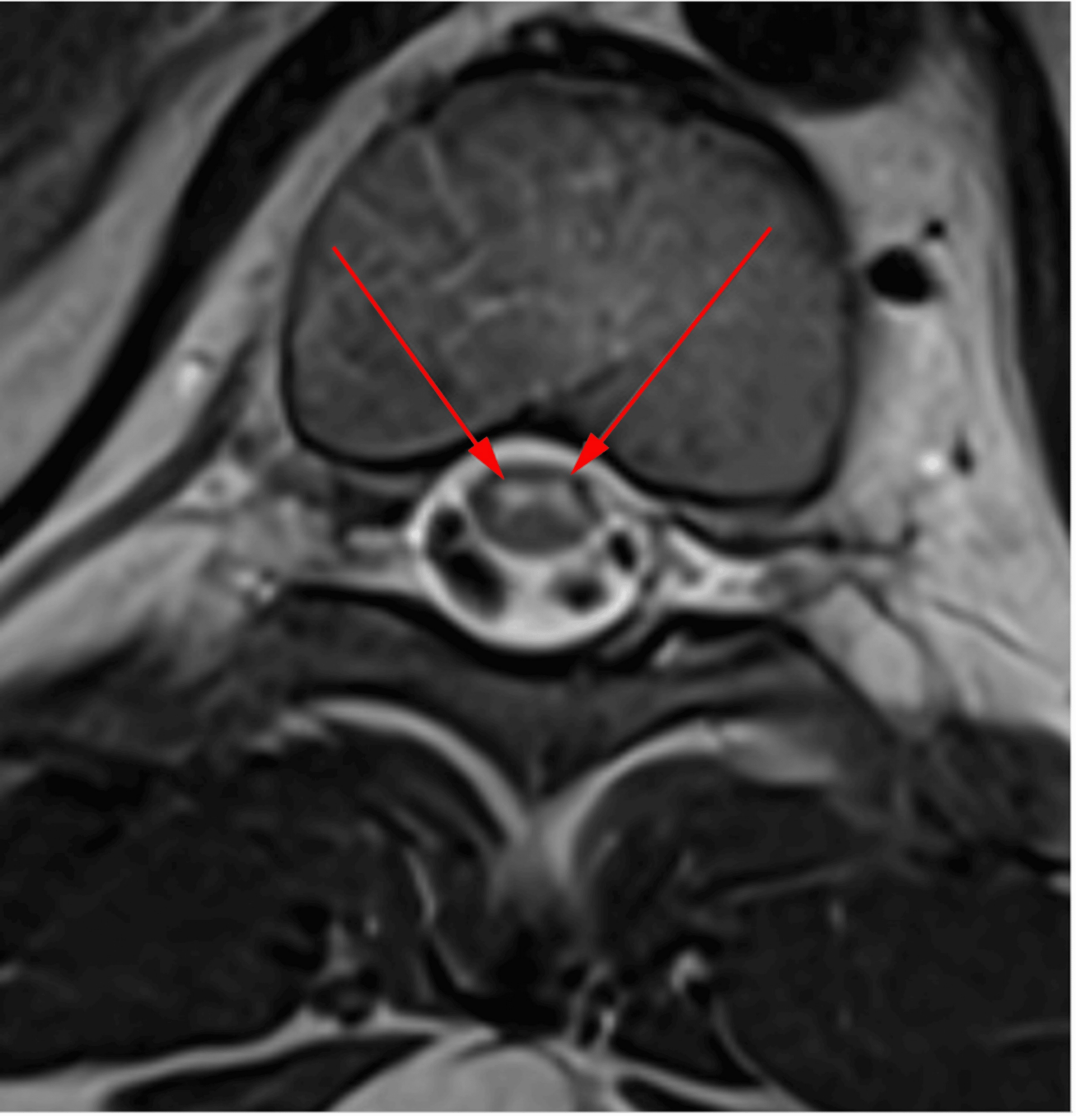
Axial T2-weighted MRI of the thoracic spine The red arrows demonstrate a hyperintense signal abnormality in the ventral spinal cord, representative of an Owl’s eye configuration.
